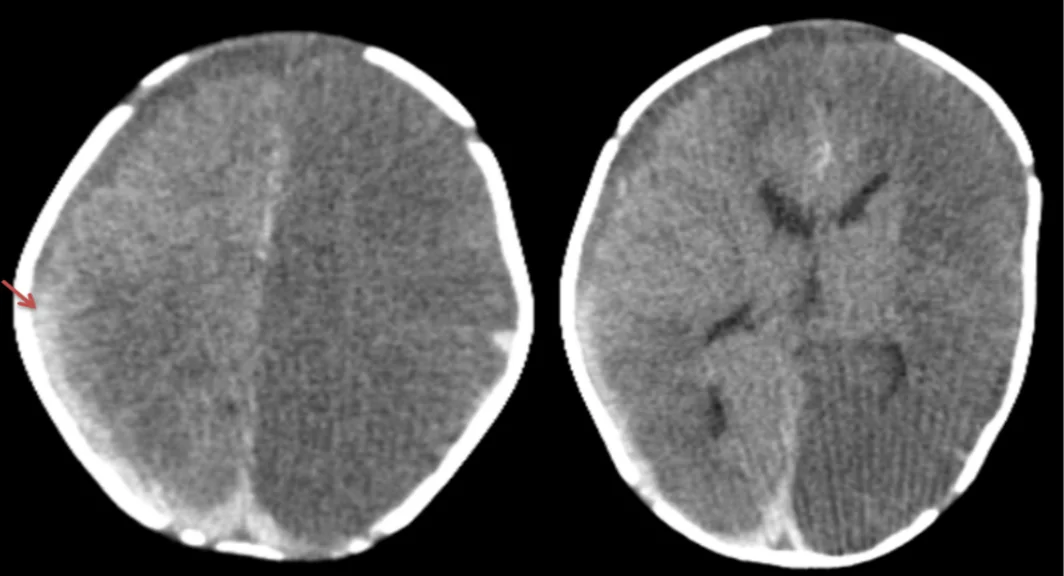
Non‐contrast axial computed tomography of the brain demonstrating diffuse hypoxic–ischemic cerebral changes with a mild right‐sided subacute subdural hematoma.
